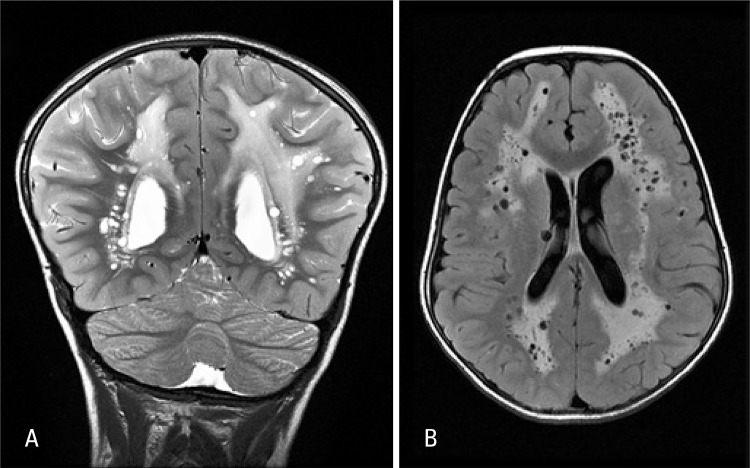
Coronal T2 weighted magnetic resonance (A) and axial fluid attenuated inversion recovery (FLAIR) (B). T2-weighted Hypersignal and FLAIR of white matter on brain hemispheres with periventricular and central predominance in almost symmetric and diffuse manner. Several cysts images spread throughout affected white matter (more linear configured at some areas), which seemed to correspond to dilatation of perivascular spaces
